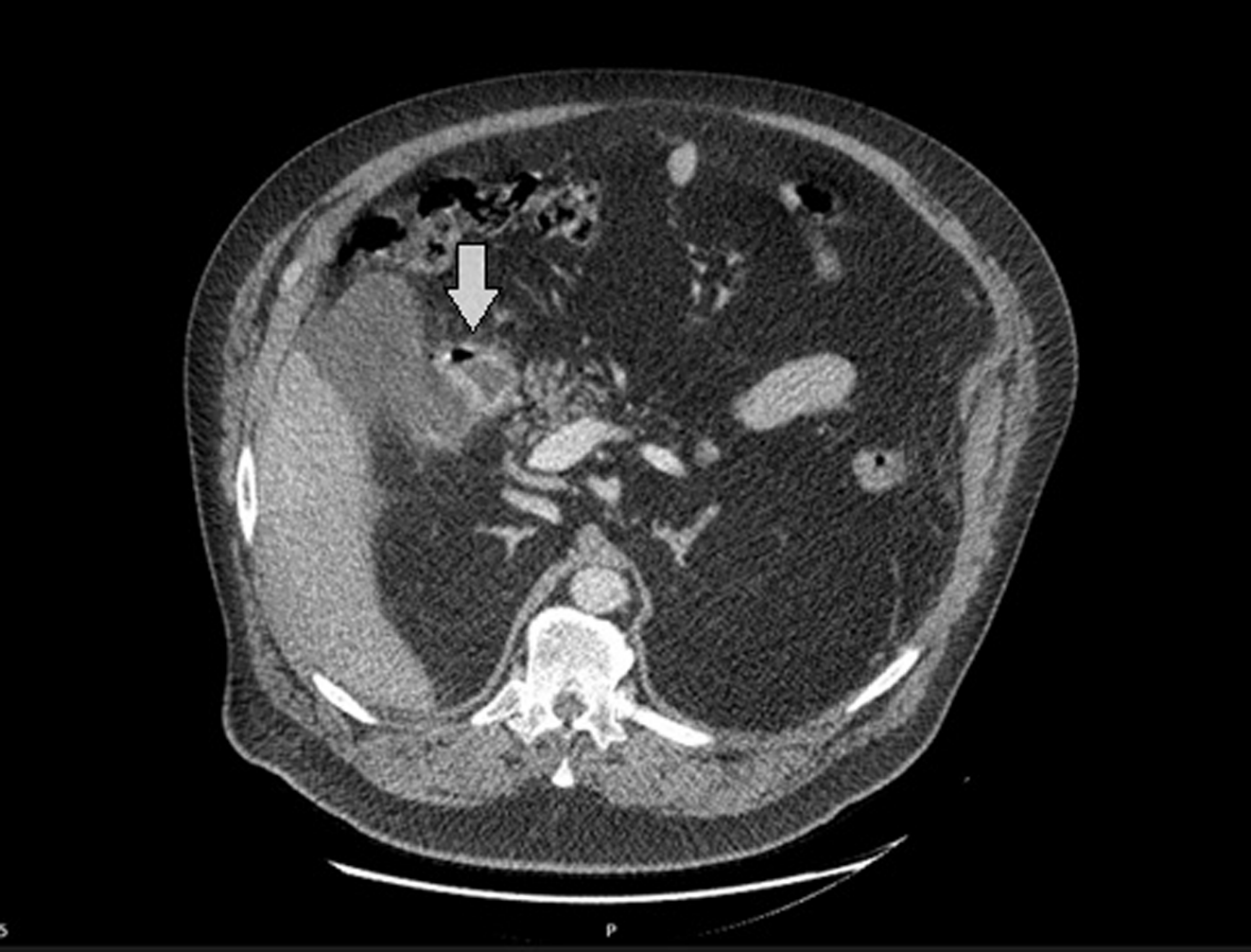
CT showed a thickened wall of the duodenum and free air.
